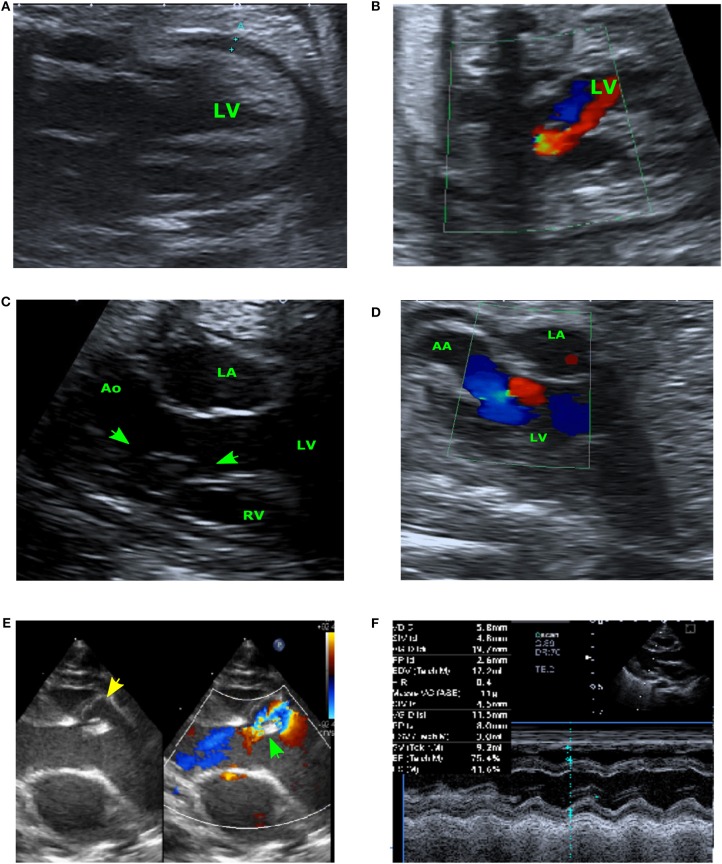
Prenatal echocardiogram at 24SG (A,B). (A) Four-chambers view showing the cardiac effusion and the enlarged LV wall. (B) Aortic flow Doppler in early systole showing anterograde LV ejection (in blue) and important regurgitation caused by ALVT (in red) –Prenatal echocardiogram at 28 SG (C,D). (C) Long-axis view showing the ALVT with its LV orifice (arrow) and its aortic orifice (star). (D) Modified aortic across view: Doppler flow of ALVT. Neonatal echocardiography (E,F). (E) Pre-operative long-axis view showing the ALVT (the left-side image shows the yellow jet of the tunnel, and the right-side image shows the aliasing flow in the ventricular extremity of tunnel); the green arrow indicates the annular aortic valve). (F) Post-operative TM-view showing the restoration of the left ventricular function after surgery.
